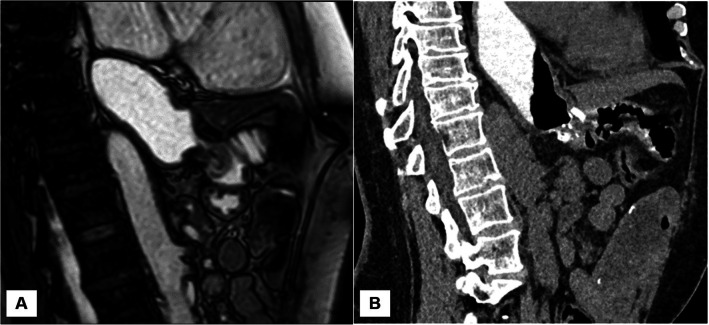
A 30-year-old female who underwent RYGB, pouch resizing, and banding; parasagittal dynamic bFFE MRI (a) and reconstructed 3D-CT (b) demonstrate the distended pouch. There is mild ITM
